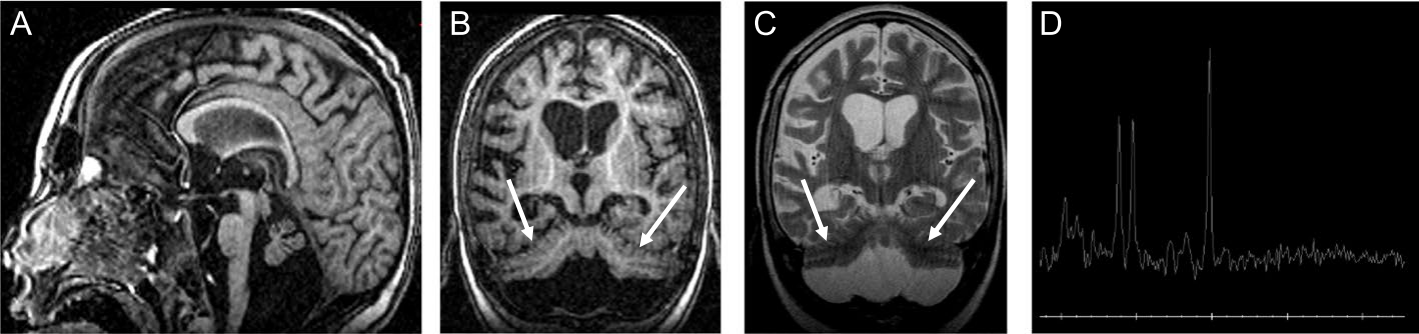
Patient brain MRI at 6 years. A Sagittal T1-weighted image showing pontocerebellar hypoplasia. B
